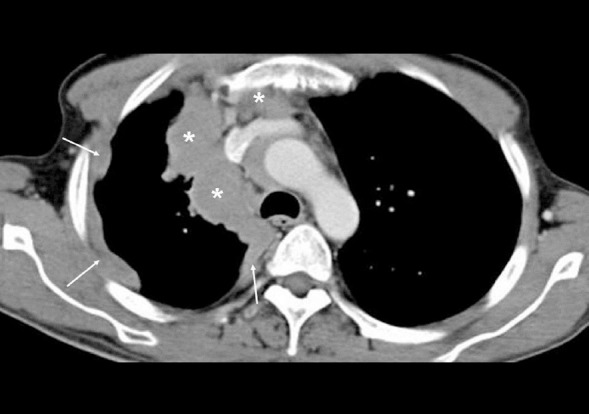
Contrast-enhanced CT of the chest of a 51-year-old male with invasive thymoma and pleural metastases is shown above. Thymoma appears as a lobulated mediastinal mass (asterisks) encircling the superior vena cava. The thickening seen around the lateral and medical aspect of the right hemithorax (arrows) represents pleural metastases.
